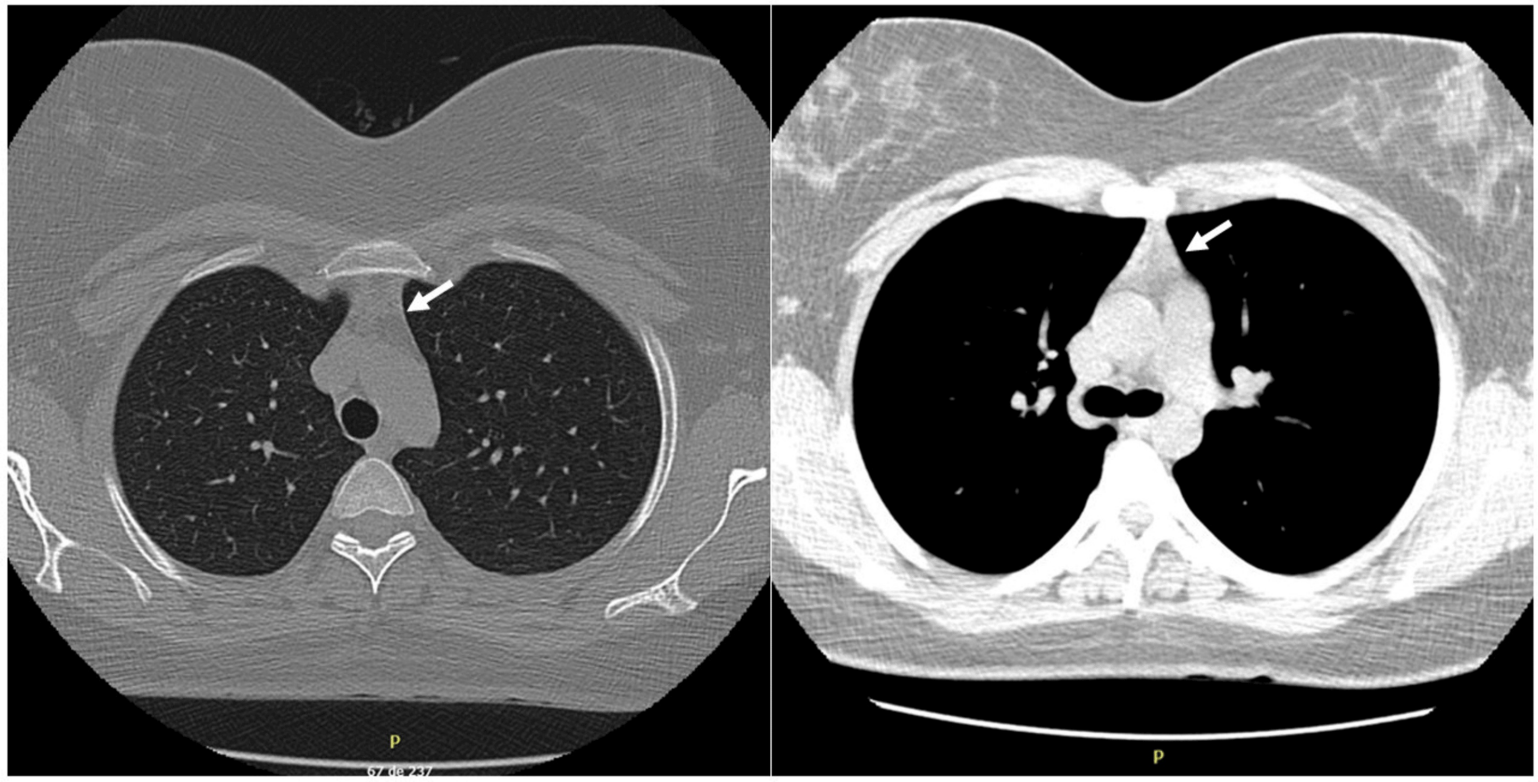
Axial non-contrast chest computed tomography (CT) scans. The left image, obtained in the bronchial phase, shows normal lung parenchyma and mediastinal anatomy without evidence of masses (white arrow). The right image, acquired in the mediastinal phase, demonstrates residual thymic tissue in the anterior mediastinum (white arrow), with no signs of thymoma. These findings are consistent with persistent thymic remnants in a patient with acetylcholine receptor antibody-positive generalized myasthenia gravis.
